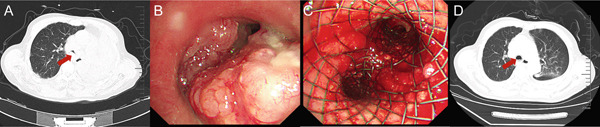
The improvement of MCAO‐related comorbidities after the therapeutic bronchoscopy. (A) Chest computed tomography (CT) revealed the right‐sided obstructive pneumonia and atelectasis secondary to stenosis of the right main bronchus (red arrow). (B) Bronchoscopy revealed a protruding mass in the right main bronchus. (C) The airway lumen was fully opened after the placement of an airway metal stent. (D) CT showed a marked improvement of obstructive pneumonia and atelectasis after metal stent placement (red arrow).
